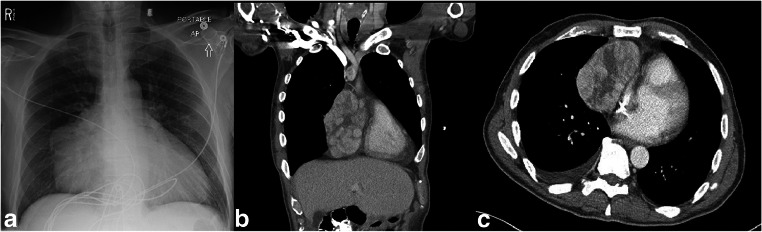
a Chest X-ray before thymectomy and thymomectomy. b, c Chest CT scan: right-sided, anterior mediastinal well-circumscribed mass without evidence of gross infiltration or necrotic, cystic, or calcified components
